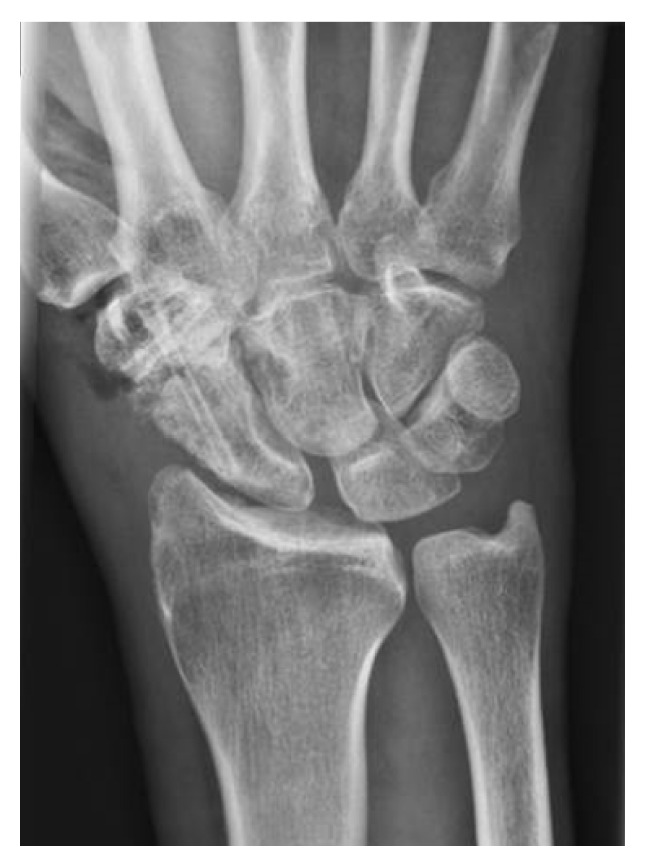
X-ray right wrist a.p. following STT-fusion with magnesium-based screws.
